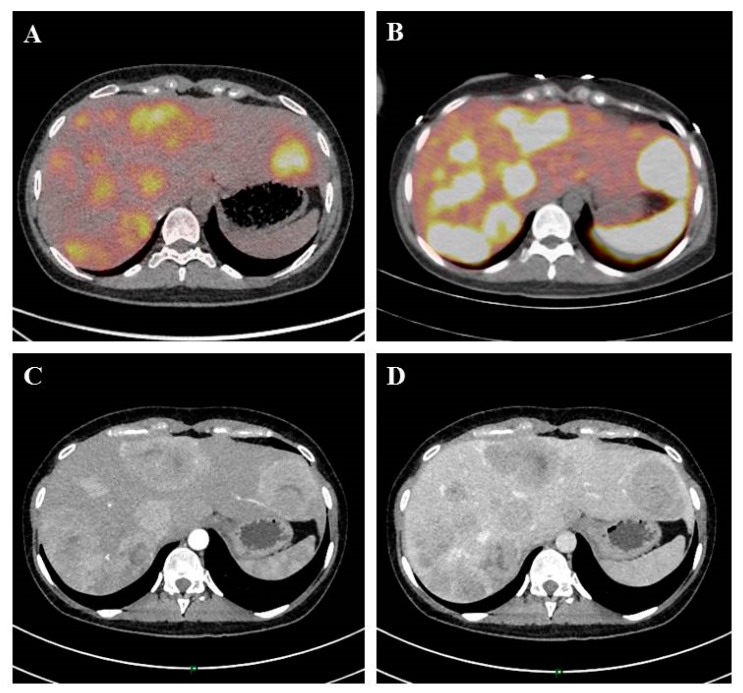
A 24-year-old patient with liver metastasis of a pancreatic neuroendocrine tumor. Initial evaluation identified liver metastases on ^18^ F-FDG PET Computed tomography scan (A), ^68^Ga-DOTATOC (B), computed tomography scan at the arterial phase (C), and computed tomography scan at the venous portal phase (D).
